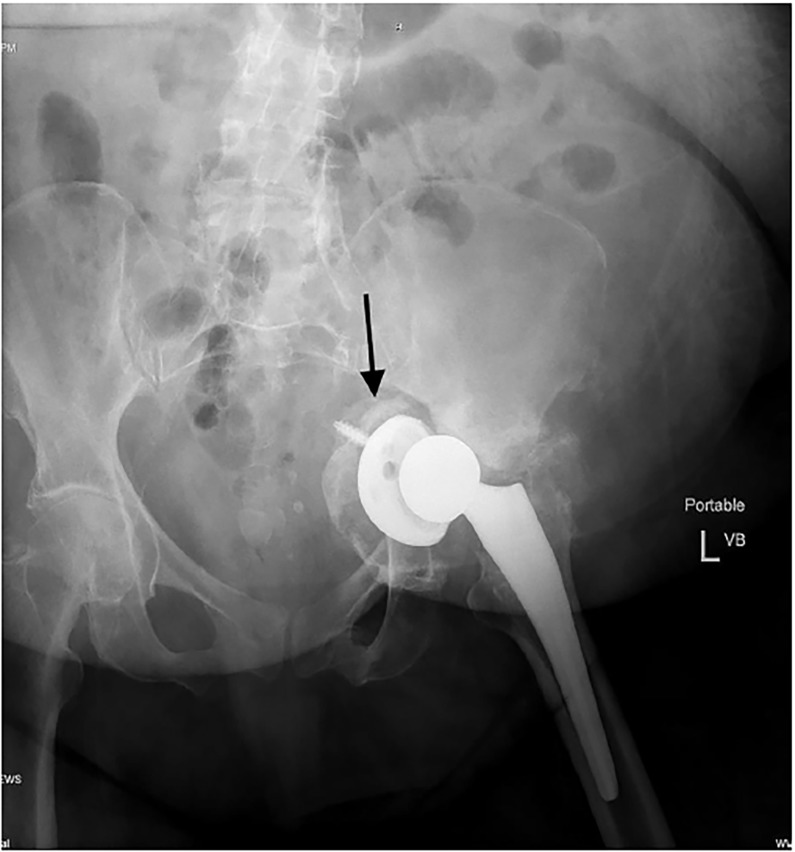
Anterior-posterior radiograph of the pelvis demonstrating medial displacement of the acetabulum and femoral head (arrow) into the lesser pelvis.
